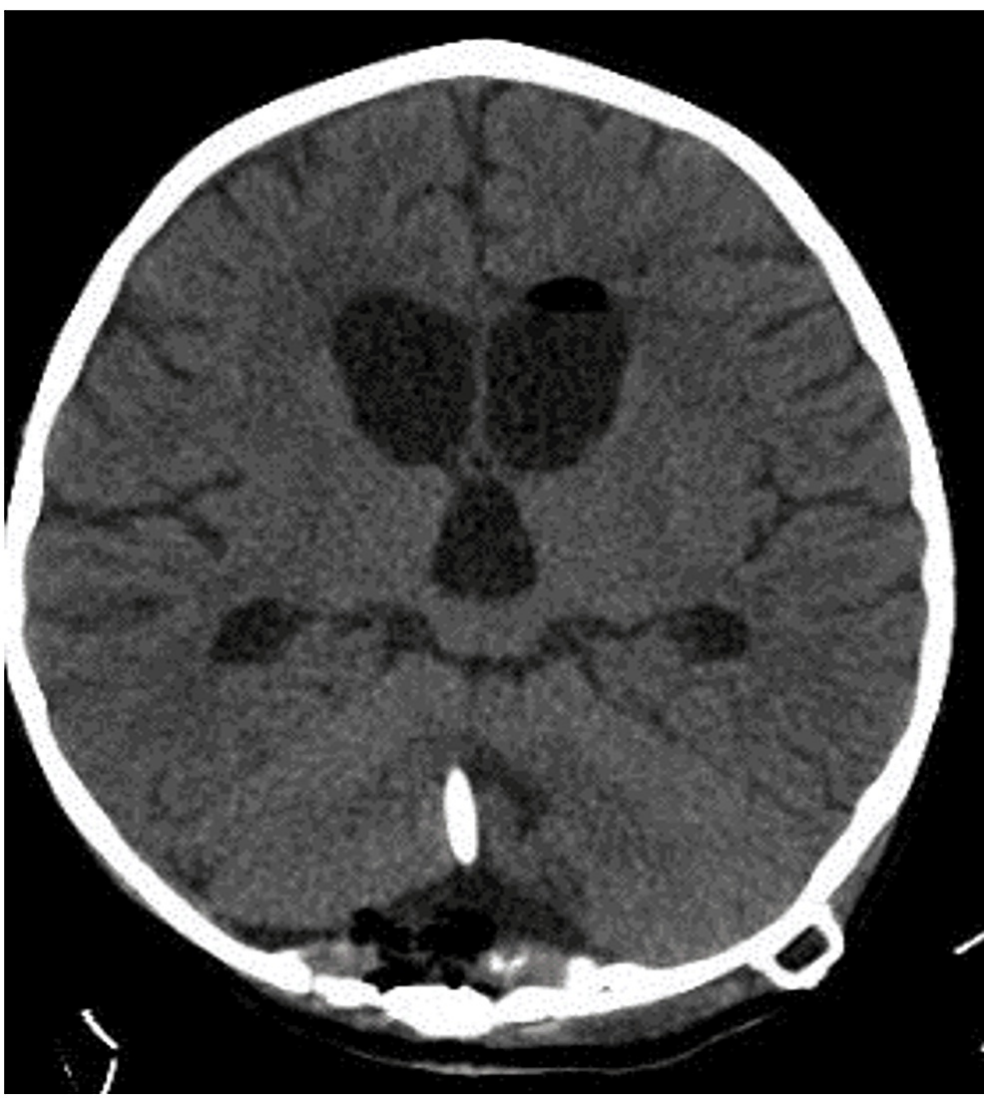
Postoperative CT brain scans. Axial cut with definitive ventriculoperitoneal shun tip and reservoir.
